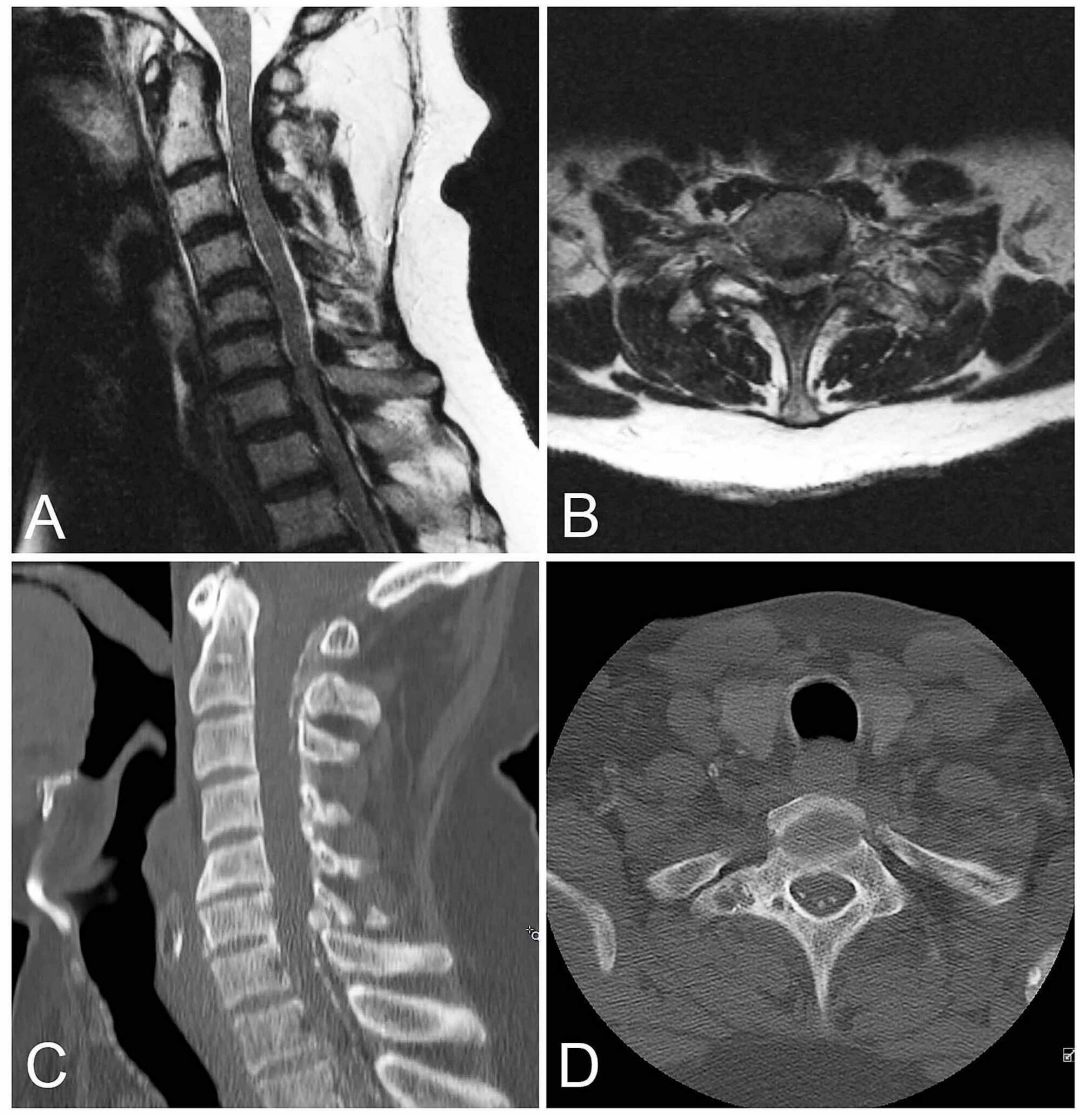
Cervical spine MRI and CT demonstrating spinal stenosis. Sagittal and axial T2 weighted MRI (A, B) of the cervical spine demonstrate severe central canal stenosis worse at C6-T1 causing mild T2 cord signal change. Sagittal and axial CT of the cervical spine (C, D) demonstrate extensive posterior calcified lesions throughout the cervical canal.
